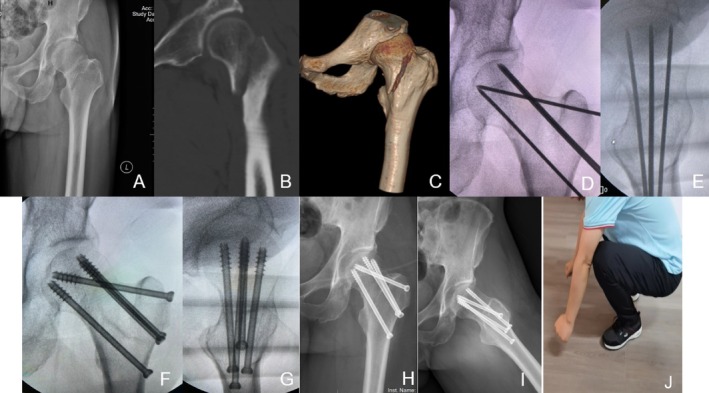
Patient, male, 47 years old, with left femoral neck fracture. (A–C) Preoperative X‐ray and CT scans revealed a Pauwels III femoral neck fracture on the left side. (D–G) Postoperative lateral X‐ray demonstrates good reduction of the fracture, and the placement of the hollow screw is satisfactory. (H–J) The last follow‐up X‐ray and the patient's hip joint functional position.
